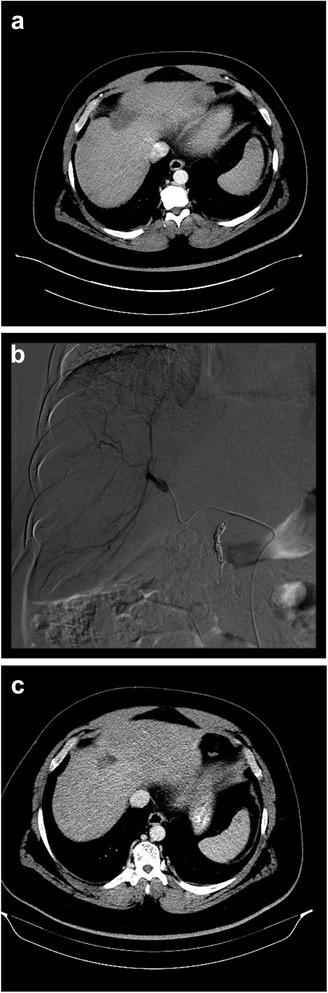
Pre, intra, and post TARE imaging (a) Pre-procedural CT demonstrating a segment 4 metastatic pancreatic adenocarcinoma measuring 4.4 x 2.2 cm (b) Hepatic angiogram demonstrating enhancing segment 4 mass with aberrant arterial vasculature (c) 3 month post procedure CT demonstrating PR to TARE with lesion now measuring 2.8 x 1.8 cm
